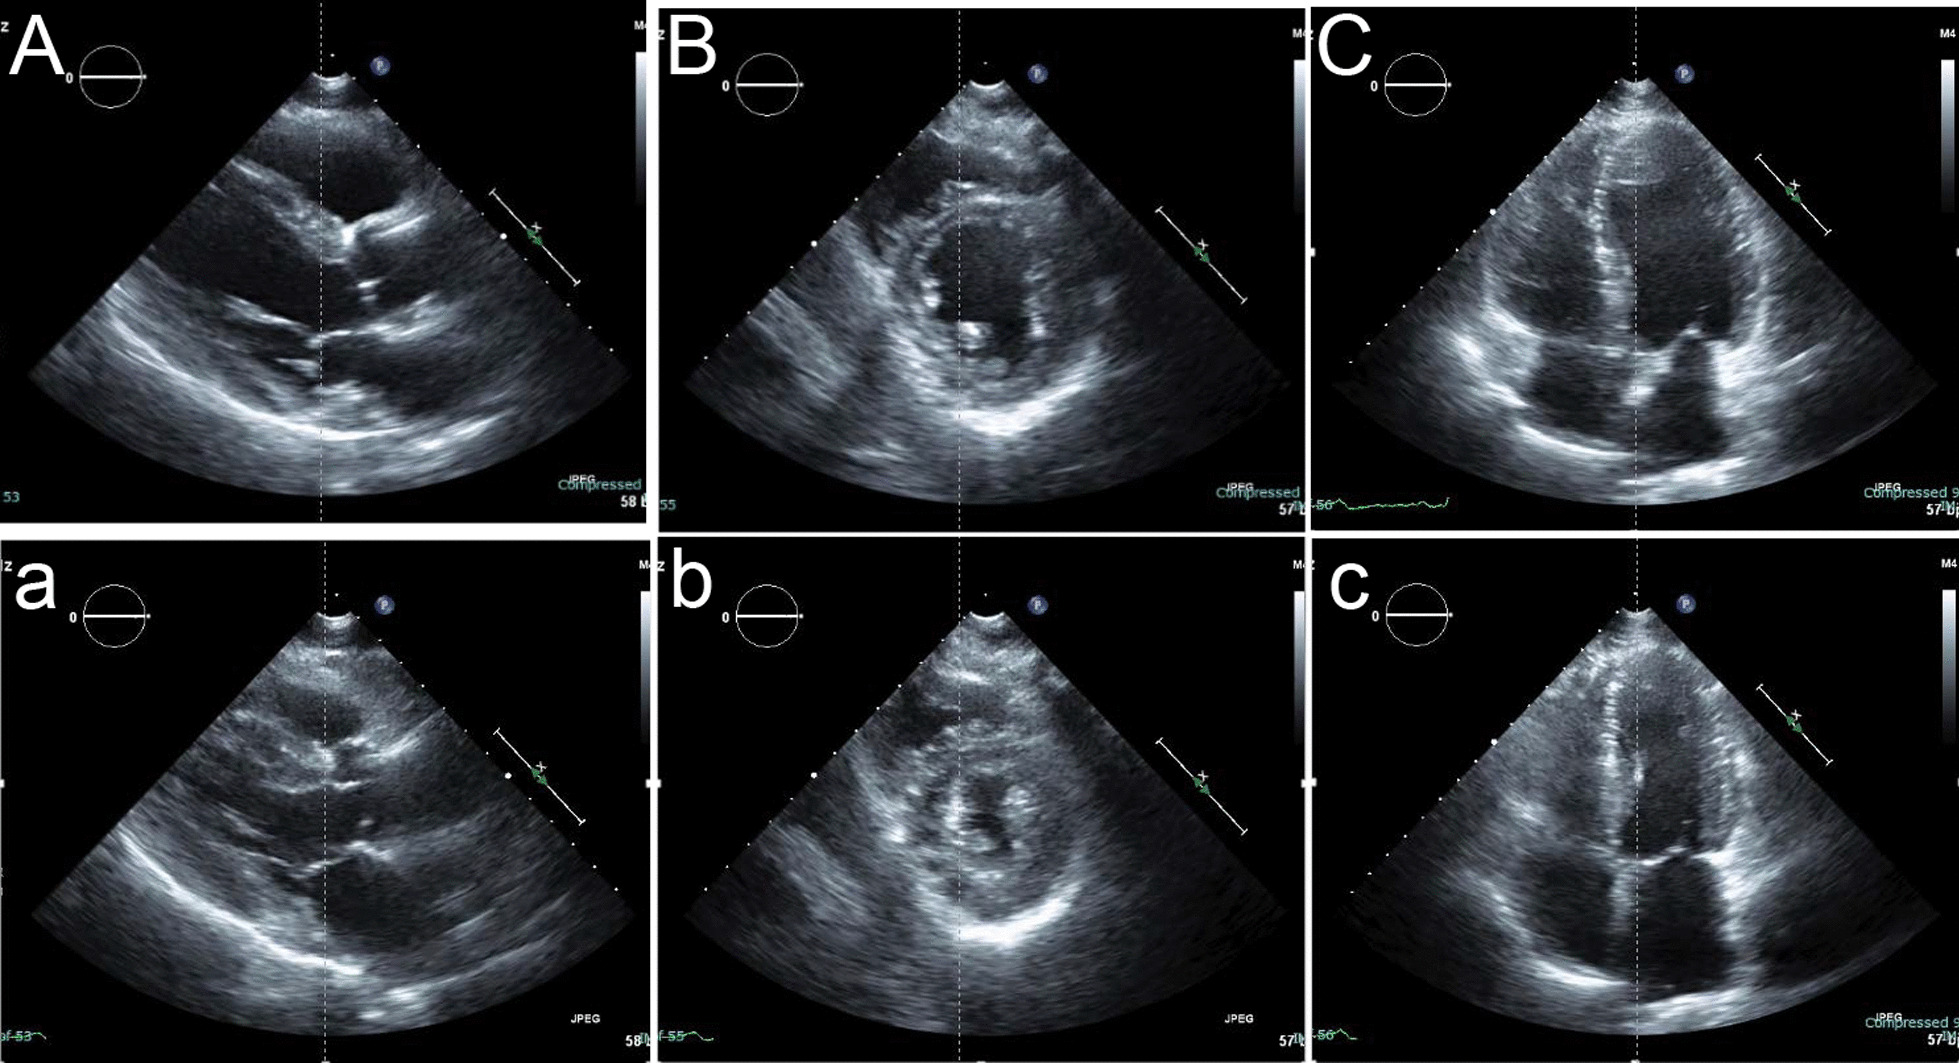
Echocardiogram obtained before myocardial infarction. Normal vetricular function is seen in the long-axis, short-axis, and four-chamber views in diastole (A, B, C, respectively) and systole (a, b, c, respectively)
